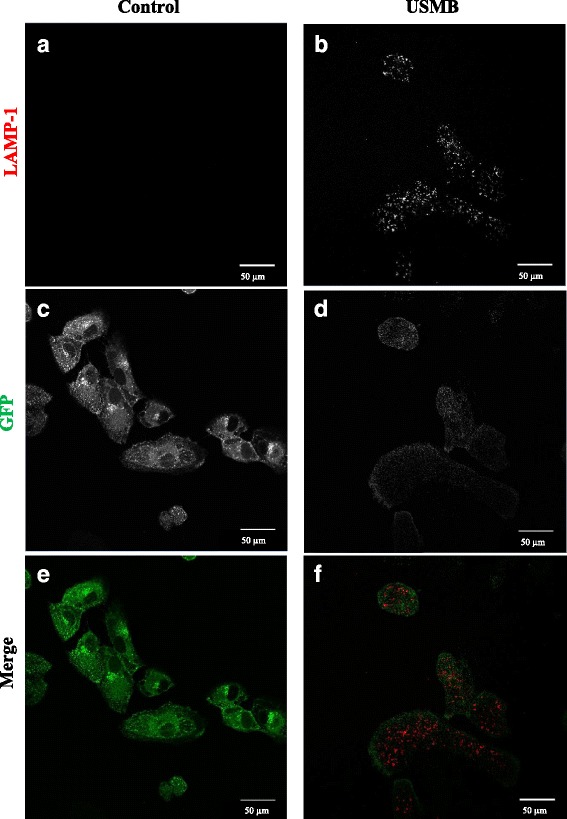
The localization of Lysosomal Associated Membrane Protein-1 (LAMP-1) antibody in Green Fluorescent Protein (GFP)-transfected Retinal Pigmented Epithelial cells (RPE). a LAMP-1 antibody binding in untreated control (b) LAMP-1 antibody binding in ultrasound and microbubble (USMB) treated cells (c) GFP-clathrin in untreated control (d) GFP-clathrin in USMB-treated cells (e) Merged channels of GFP-clathrin (*green*) and LAMP-1 antibody (*red*) in untreated control (f) Merged channels of GFP-clathrin and LAMP-1 antibody in USMB-treated cells (*n =* 3). Scale bar = 50 μm
